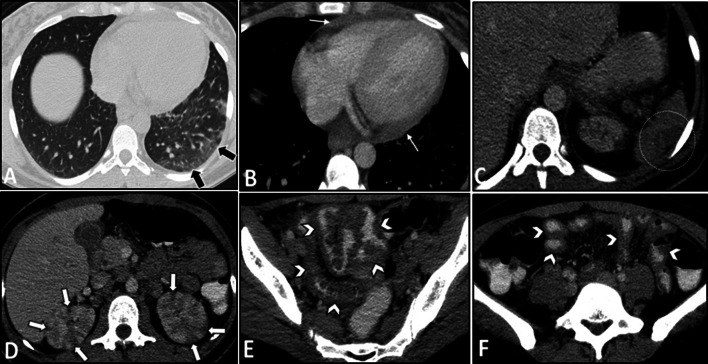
Intestinal vasculitis and renal infarction in a 29-year-old female with known SLE presenting with fever, malaise, generalized abdominal pain, dyspnea, and elevated ESR. An abdominopelvic CT scan with IV and oral contrast was done. There are subpleural patchy areas of GGO at the left lower lobe suggestive of lupus pneumonitis (thick black arrows in A). Small pericardial effusion and thickening (thin white arrows in B) are seen in favor of serositis. There is a wedge-shaped subcapsular hypodensity in the spleen (white dotted oval in C), indicative of splenic infarction. Multiple bilateral wedge-shaped renal parenchymal infarcts are also depicted (thick white arrows in D). Diffuse circumferential wall thickening of ileal loops (white arrowheads in E and F) is evident, representing lupus enteritis
